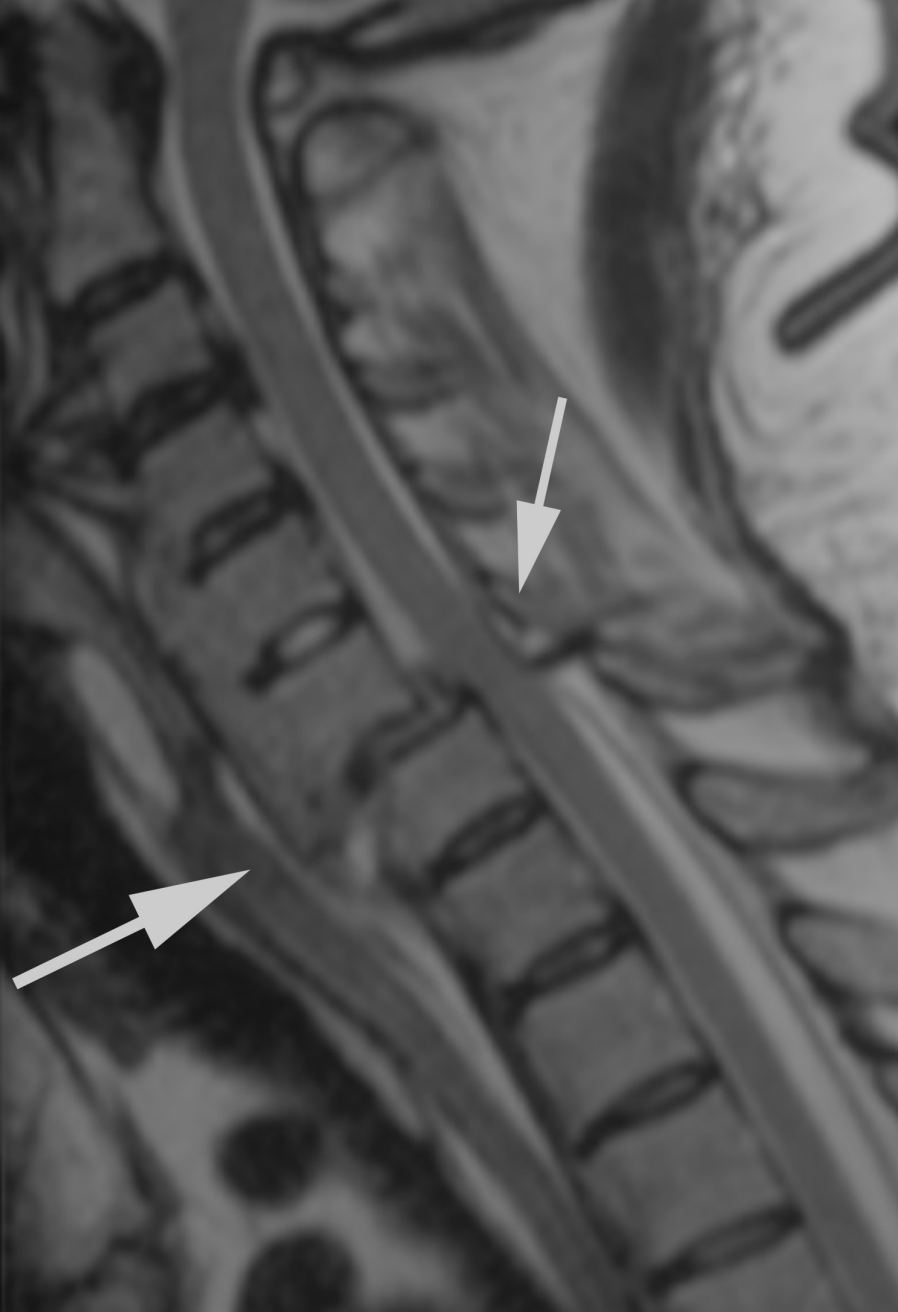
T2-weighed MRI scan in a median-sagittal plane of the cervical spine. There is a C 6/7 luxation fracture without evidence for a profound spinal cord lesion. The degenerative alterations, particularly the large bridging spondylophytes are consistent with Forestier's disease.
